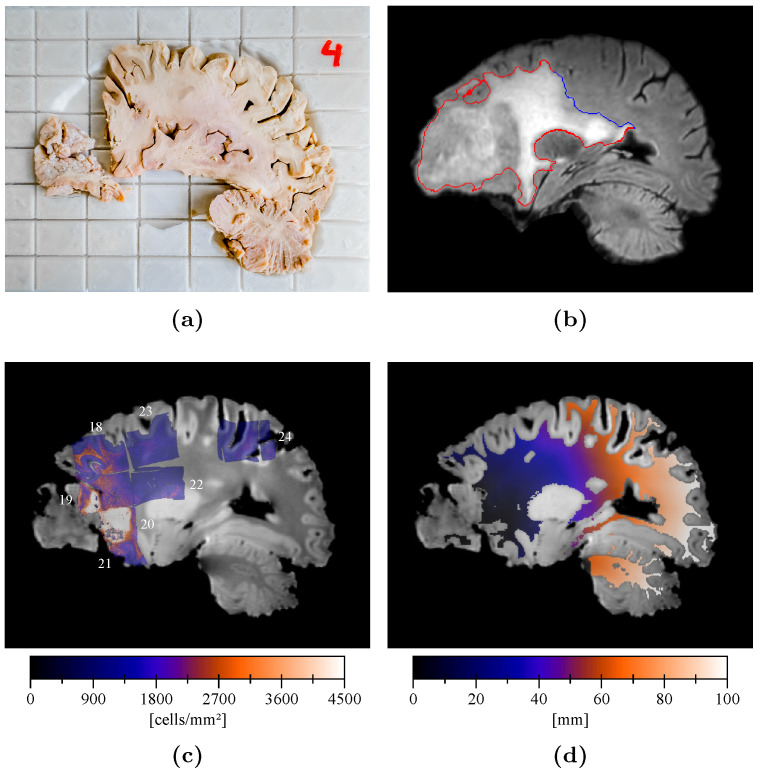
Cell density profile analysis. (a) Brain slice inside its 3D-printed cutting guide. (b) Corresponding slice of the registered in vivo T2-FLAIR image with segmented edema outlines. The blue and red segments of the outline respectively correspond to free and non-free to diffuse parts of the edema boundary (see Section 4). (c) Corresponding slice of the ex vivo T1 image (grayscale) and superimposed registered cell density maps (colored) with their slide number (see Table A1). (d) Corresponding slice of the geodesic distance map to the tumor core across white matter.
